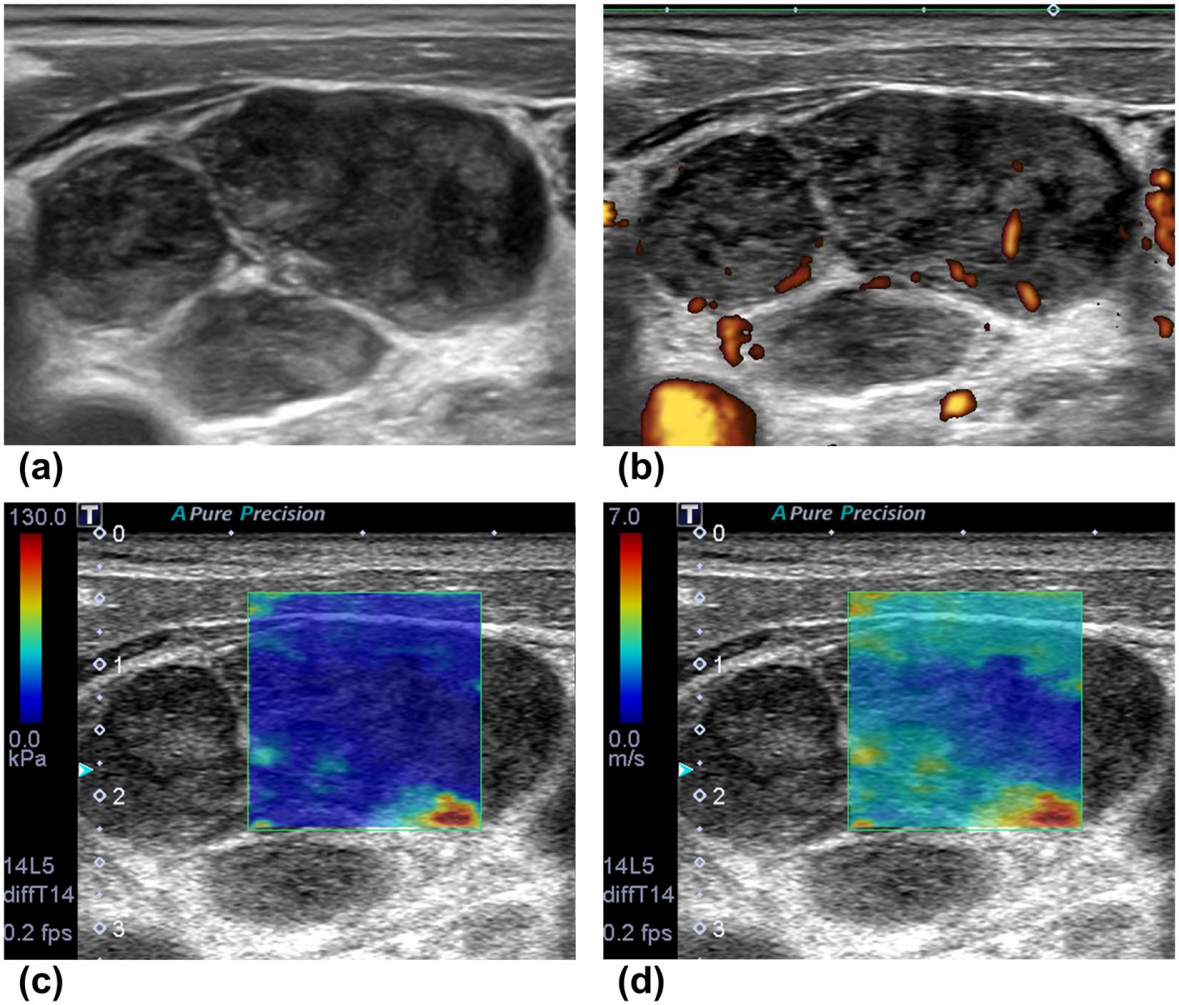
The case of diffuse large B cell lymphoma. On conventional ultrasound (a: 2D echo, b: power Doppler US), multiple round lymph nodes have heterogeneous echogenicity and show indistinct border with internal mixed vascularity. Two readers diagnosed these lymph nodes as metastasis with 2D and Doppler US. After evaluation with shear wave elastography (c: the elasticity map, d: the speed map), all readers classified the nodes as pattern 2, and the two readers corrected their diagnosis as lymphoma. Maximum elasticity, relative elasticity, maximum speed and relative speed were 30.4 kPa, 1.32, 3.16 m/s and 1.16, respectively.
